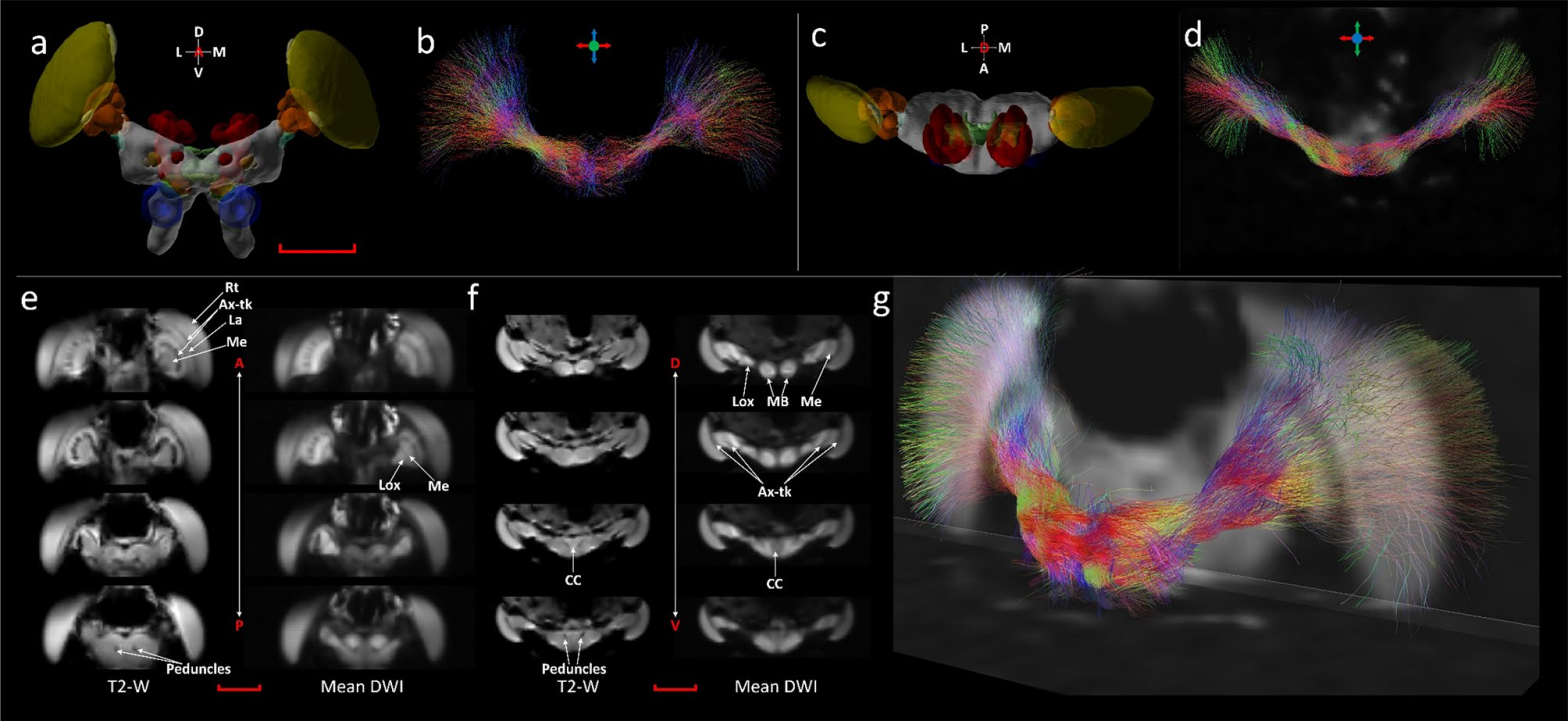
Anatomical regions and tract connectivity derived from dMRI in a live locust. (a) Anterior view of 3D anatomical representation taken, with permission, from http://insectbraindb.org^44^. (b) 3D projections of directionally encoded tracts from synaptic neuropils (coronal slice, anterior view). (c) Dorsal view of 3D anatomical representation taken, with permission, from http://insectbraindb.org^44^. (d) 3D projections of directionally encoded tracts from synaptic neuropils (axial slice dorsal view). (e) Coronal slice (anterior to posterior view) using T2-W and averaged DWI based contrasts. (f) Axial slice (dorsal to ventral view) using T2-W and averaged DWI based endogenous contrasts. (g) 3D view of axonal tract projections from synaptic neuropils. In (b) and (d) coloured crosshairs highlight the direction of tracts. In (e) and (f) T2-W maps, regions with longer transverse relaxation (T2-relaxation) are hyperintense and regions exhibiting shorter transverse relaxation are hypointense. In averaged DWI based maps, regions of low diffusivity are hyperintense and regions of high diffusivity are hyperintense. *Ax-tk* axonal tracts, *CC* central complex, *La* lamina, *Lox* lobula complex, *MB* mushroom body, *Me* medulla, *P* peduncles, *Rt* retina. Scale bar 500 µm.
